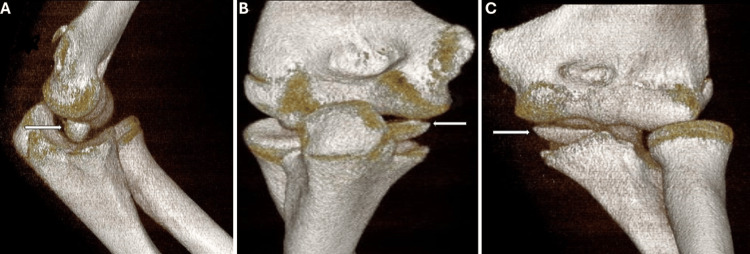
3D reconstruction on CT of the left elbow (lateral, posterior, and anterior view) A: Lateral view showing a fracture-avulsion of the epiphyseal nucleus of the epicondyle with dislocation of the fragment at the intra-articular level between the medial humeral condyle and the ulna (arrow). B: Posterior view showing a fracture-avulsion of the epiphyseal nucleus of the epicondyle with dislocation of the fragment at the intra-articular level between the medial humeral condyle and the ulna (arrow). C: Anteroposterior view showing a fracture-avulsion of the epiphyseal nucleus of the epicondyle with dislocation of the fragment at the intra-articular level between the medial humeral condyle and the ulna (arrow).
